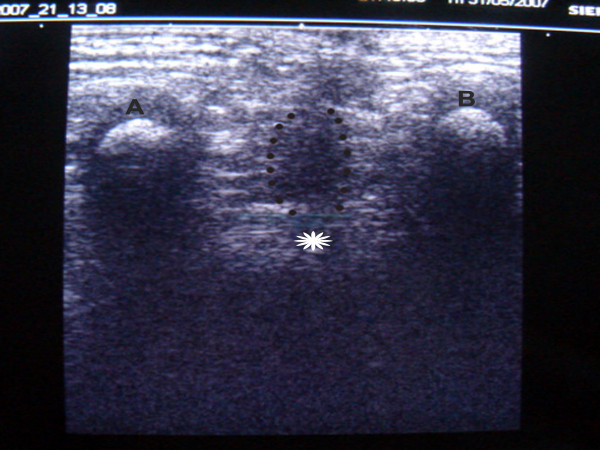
Sonogram showing important anatomical landmarks which guide accurate central placement of guide wire. Points A and B indicate ischial tuborosities. Dashed curved line indicates the medial borders of the levators on both sides. The star in the center corresponds to the position of the anal dimple.
